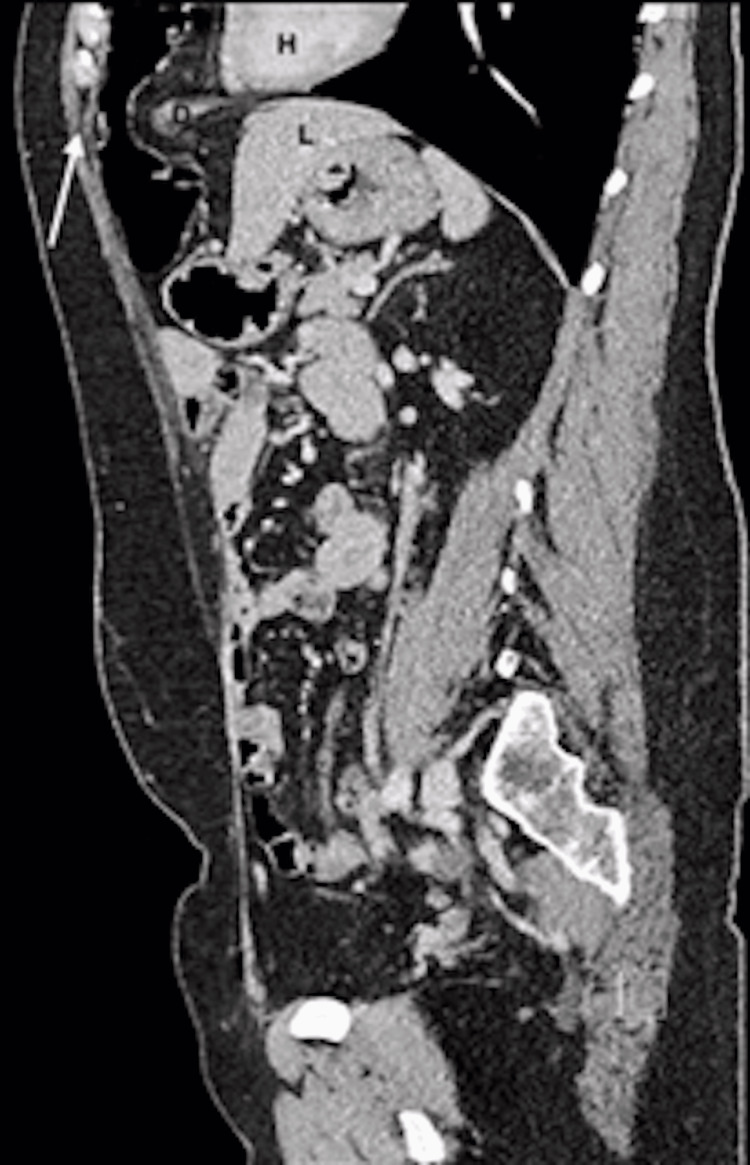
Sagittal view of abdominal and thoracic CT scan The white arrow points to the large left anterior diaphragmatic hernia with the passage of the transverse colon. H: Heart, D: Diaphragm, L: Liver
